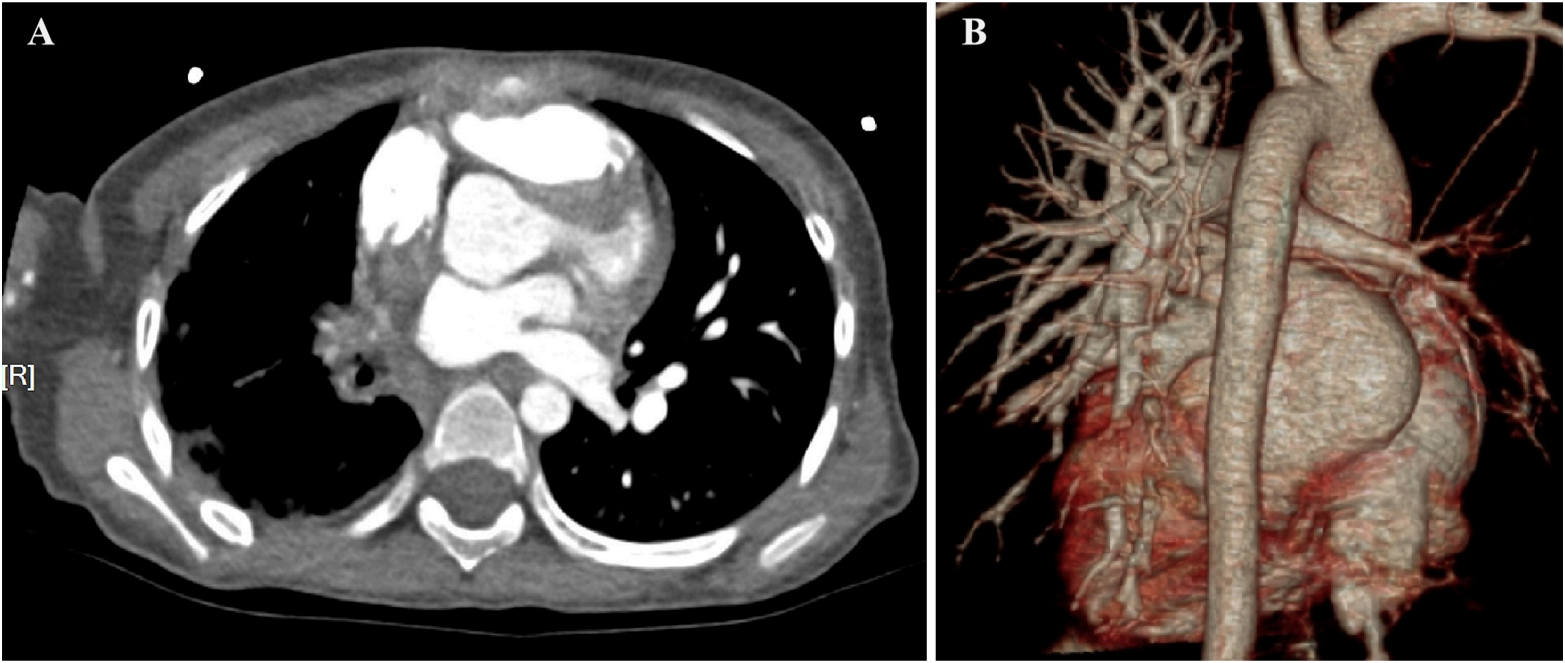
A) Cardiac CTA (mediastinum window) and B) three-dimensional vascular reconstruction of a 4-year and 11-month-old girl at her first admission (Case No. 81), both showing the absence of the right pulmonary veins, suggesting right pulmonary vein atresia. CTA = computed tomography angiography.
